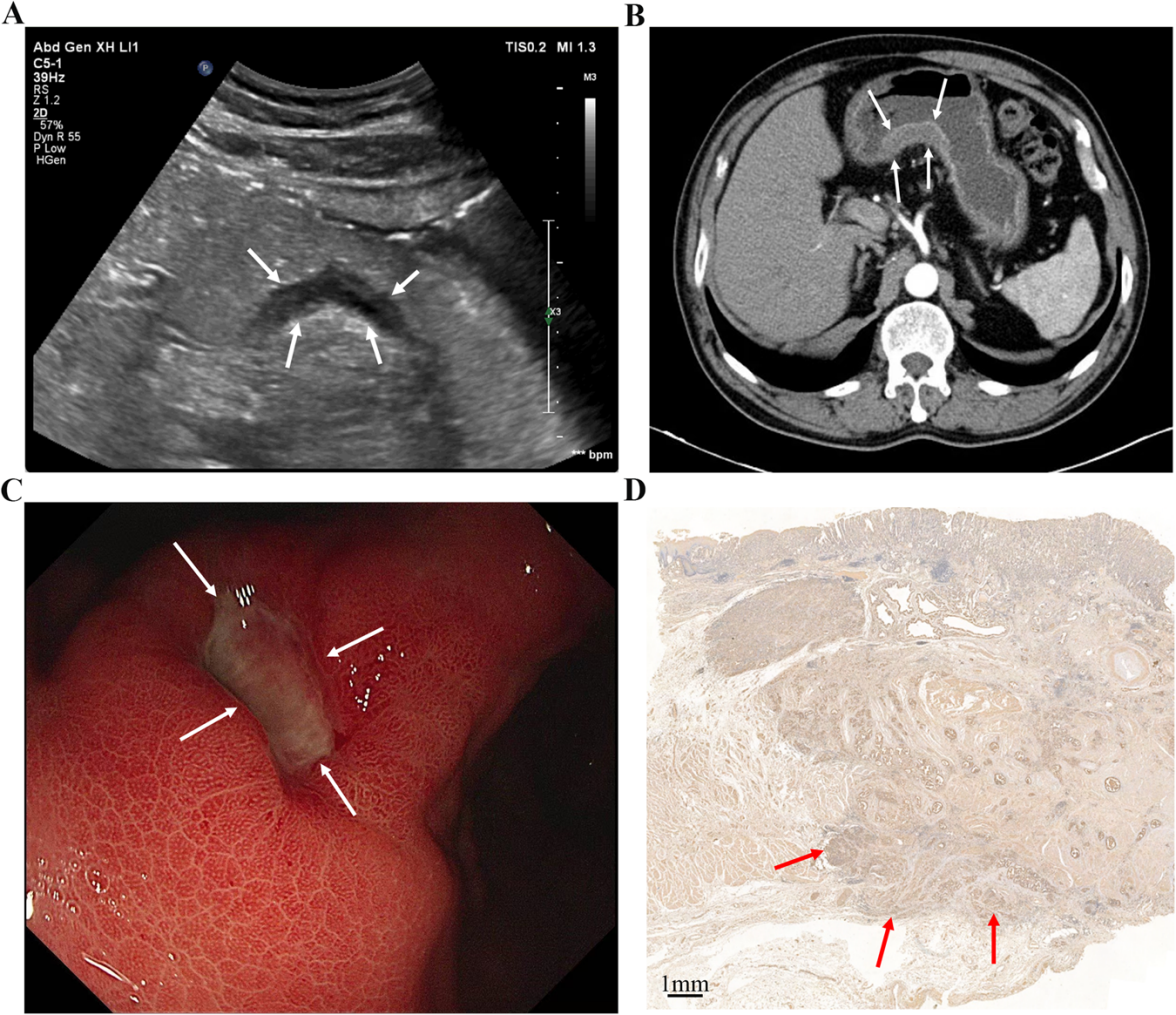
A An approx 70-year-old man underwent gastric oral contrast ultrasonography, revealing that low echo invaded the subserosal layer (cT3) at the gastric angle. The hierarchical structure of the gastric wall was unclear. The mucosa presented as rough, and the strong echogenic zone of the serosa was not smooth. B The tumor showed local thickening and enhancement, indicating invasion to the subserosal layer (cT3) of the lower part of the gastric body on enhanced computed tomography. C Electronic gastroscopy found a mucosal lesion in the gastric angle, with central ulcer formation. D The pathological result showed that the tumor invaded the muscular layer of the gastric wall to the surrounding adipose tissue, not involving the serosa (pT3) (scale bar: 1 mm)
